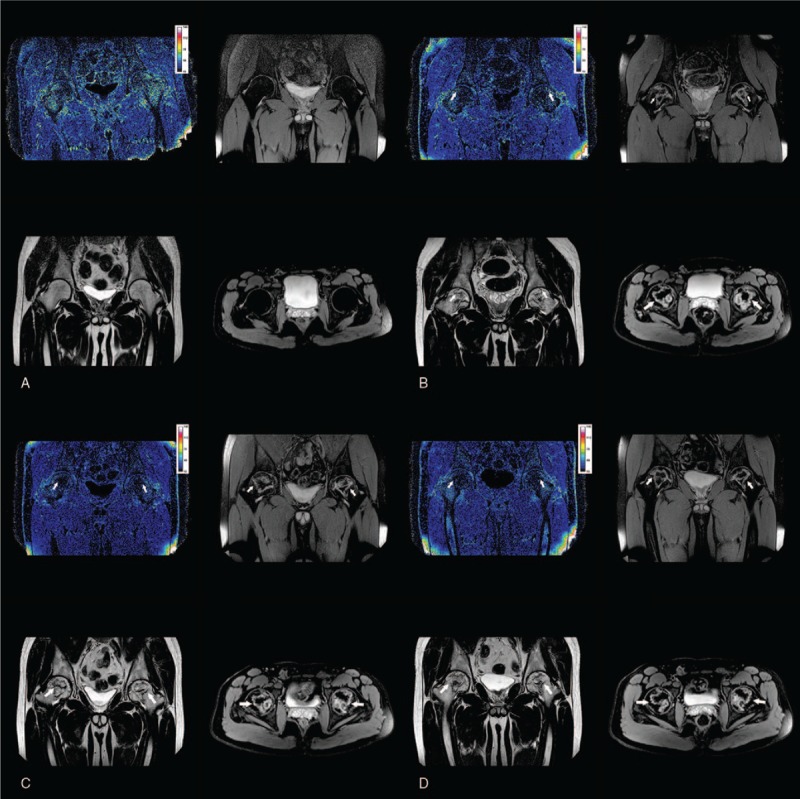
Dynamic changes in the R2∗ value and conventional MRI representation of femoral head over 4 examinations of the same patient. (A) The MRI findings at 1 month after steroid treated, which showed a slight increase of R2∗ value of the femoral head. However, the conventional MRI (coronal PDW/SPAIR, coronal T2WI/TSE, and axial T2WI/SPAIR) appeared normal. (B) The MRI findings at 4 months after steroid treated, which showed that a drop in R2∗ value of the femoral head. The lesion with a “double-line” sign on the T2WI sequence, the “serpentine-like” geographic border on the SPAIR sequence with conventional MRI. (C) The MRI findings at 7 months after steroid treated, which showed that a further decrease in R2∗ value of the femoral head. And the increase of “serpentine-like” geographic border on the SPAIR sequence with conventional MRI. (D) The MRI findings at 12 months after steroid treated, which showed that the R2∗ value of the femoral head decreased again to the minimum. And on the SPAIR sequence the “serpentine-like” geographic border grew up to the maximum size. R2∗ = total transverse relaxation time
